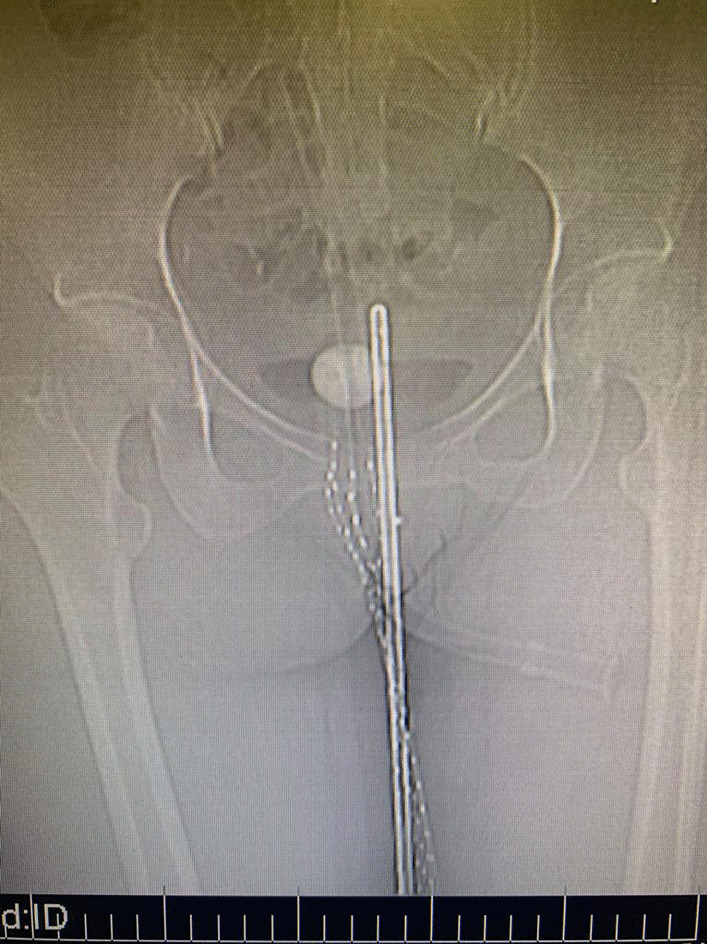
The pelvic anteroposterior plain radiography of the patient after interstitial catheter insertion
